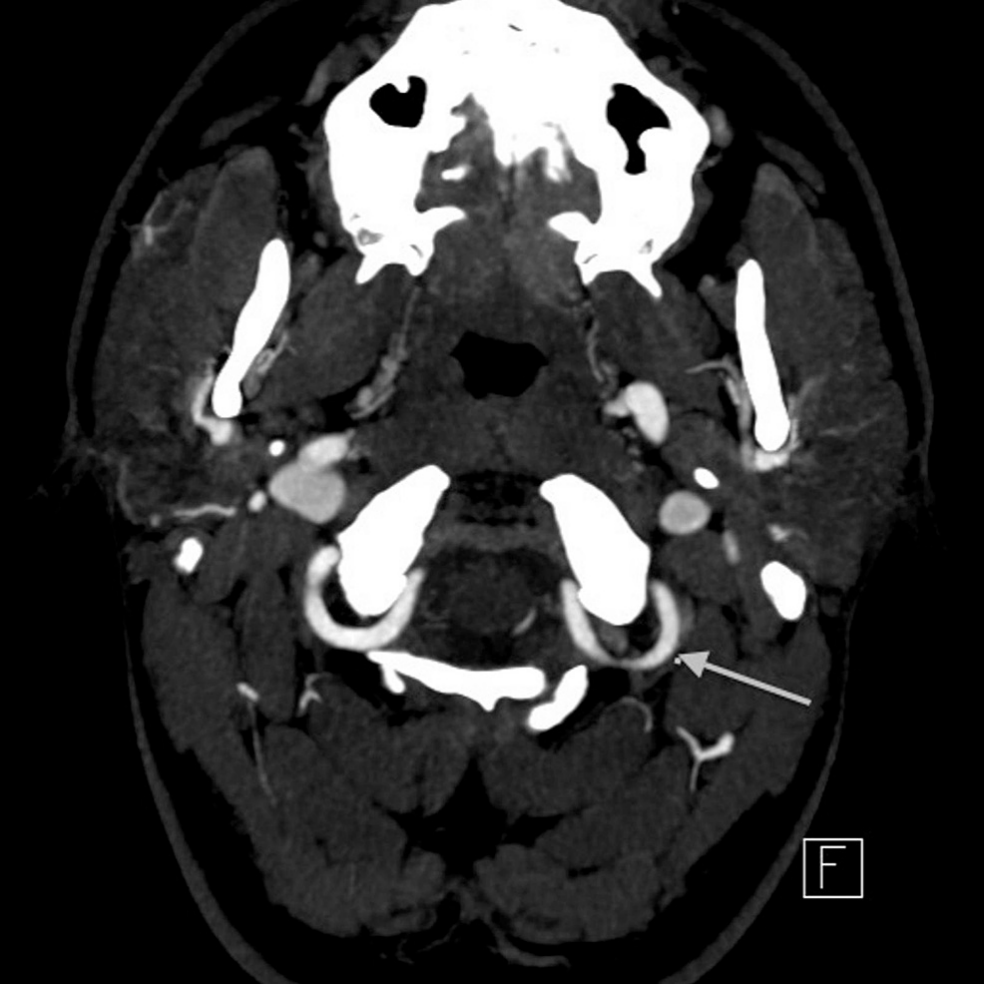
CT angiography (CTA) axial view shows severe narrowing of the left vertebral artery at C2 level with possible dissection (arrow).
